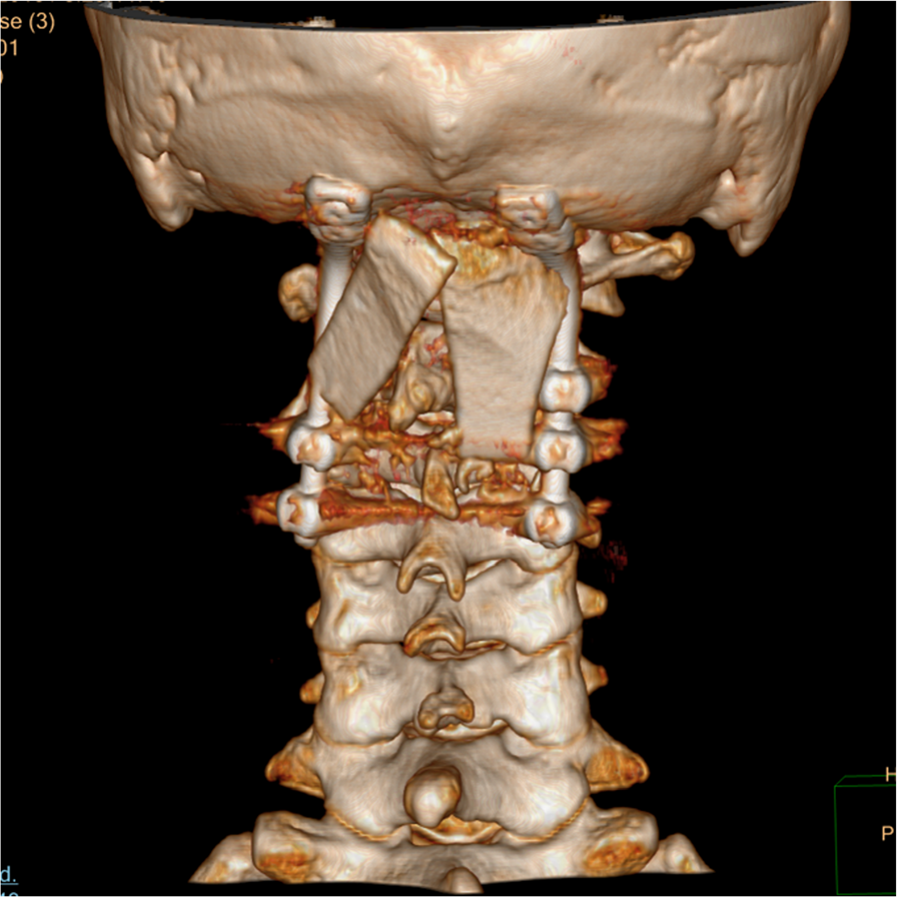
Posterior 3D-CT examination 5 years after operation showed that the internal fixation and bone graft position was good
